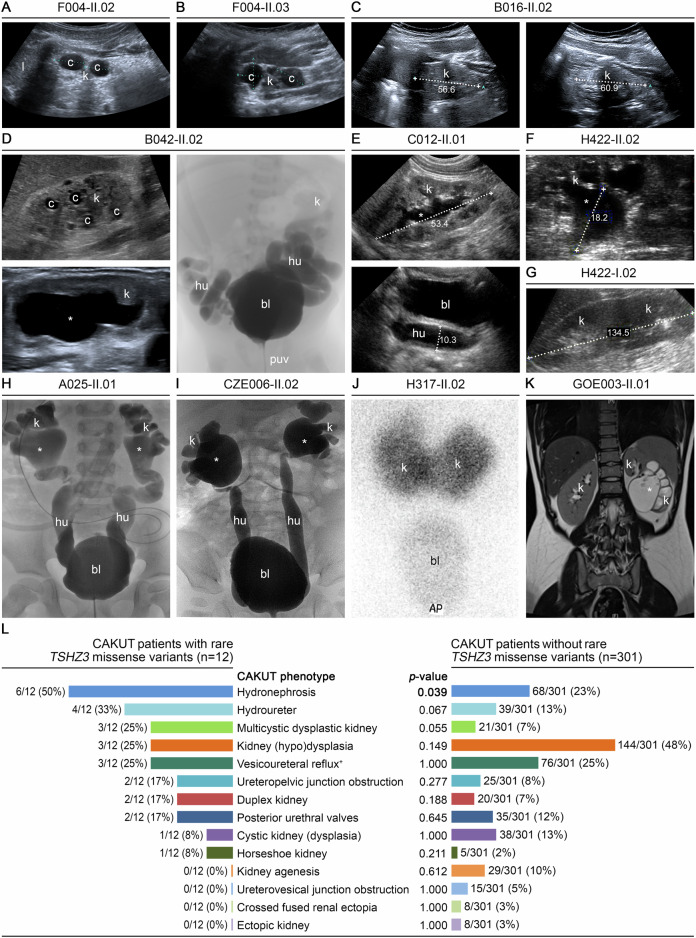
CAKUT phenotypes of patients carrying *TSHZ3* variants, and comparison of CAKUT phenotype frequency in patients with and without *TSHZ3* variants. A Patient II.02 from index family F004 was diagnosed with right-sided MCDK by ultrasound (US). B Kidney US of his brother, F004-II.03, who presented with left-sided MCDK. C Patient B016-II.02 showing hypodysplasia of both kidneys by US. D Kidney US revealed right-sided MCDK and left-sided hydronephrosis in patient B042-II.02. Voiding cystourethrography (VCUG) showing bilateral hydroureters and posterior urethral valves. E Kidney US of patient C012-II.01 showing left-sided hydronephrosis and hydroureter. F Patient H422-II.02 was diagnosed with left-sided hydronephrosis, as shown by US, and ureteropelvic junction obstruction. G Kidney US of his mother, H422-I.02, showing right-sided non-obstructive duplex kidney. H By kidney US (not shown) and VCUG, patient A025-II.01 was diagnosed with bilateral kidney hypodysplasia, hydronephrosis, hydroureters, and VUR grade IV. I VCUG of patient CZE006-II.02 revealing bilateral hydronephrosis, hydroureters, VUR grade IV–V, and posterior urethral valves. J A dimercaptosuccinic acid kidney scan in patient H317-II.02 showing a horseshoe kidney. K Magnetic resonance imaging of patient GOE003-II.01 revealing left-sided duplex kidney, kidney dysplasia of the lower pole, hydronephrosis, and ureteropelvic junction obstruction of the lower kidney. L Frequency of CAKUT phenotypes (colored bars) in patients with rare heterozygous *TSHZ3* variants (*n* = 12) compared to CAKUT patients without *TSHZ3* variants (*n* = 301). Hydronephrosis (*p* = 0.039), hydroureter (*p* = 0.067), and MCDK (*p* = 0.055) were (significantly) more frequent in CAKUT patients with versus without *TSHZ3* variants. ^*+*^ VUR was never isolated, but occurred in conjunction with structural kidney or urinary tract defects in patients analyzed here, * hydronephrosis, AP anterior posterior, bl bladder, c cyst, hu hydroureter, k kidney, l liver, puv posterior urethral valves, VUR vesicoureteral reflux.
